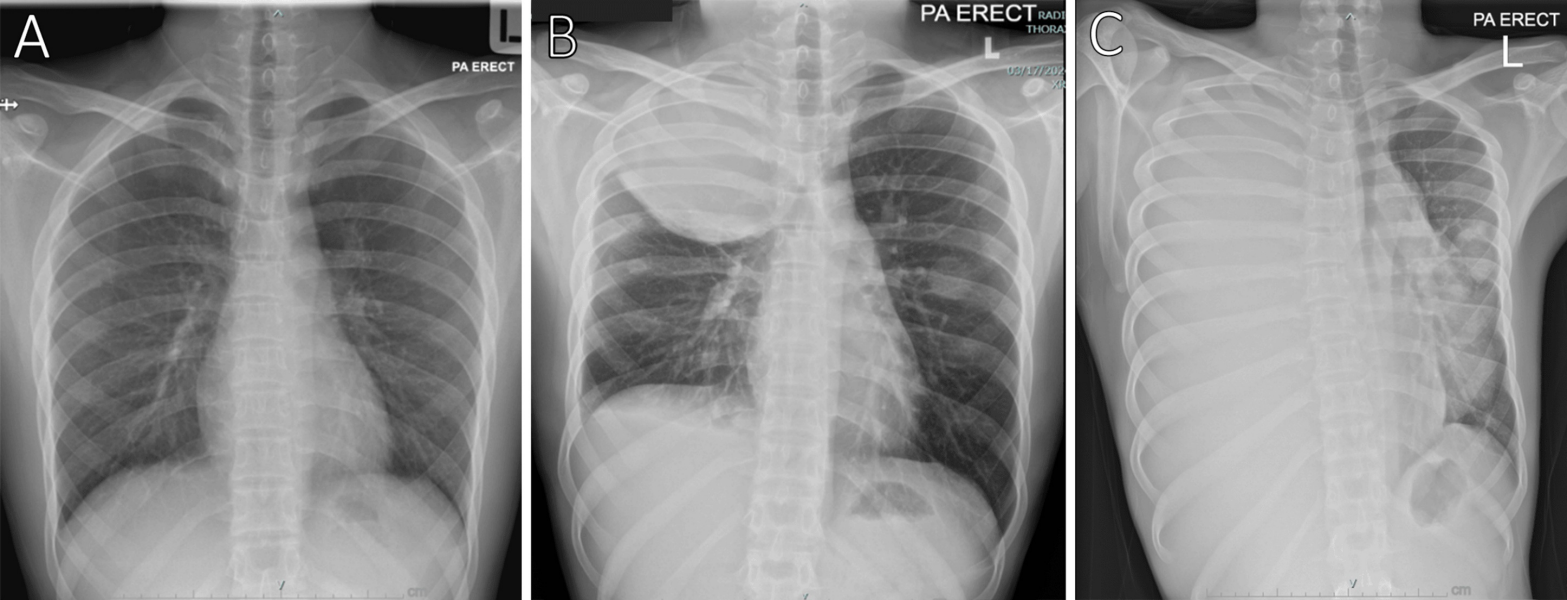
Serial chest radiography showing the rapid progression of the lung tumour upon first encounter (A), three weeks later (B), and seven weeks later (C).
